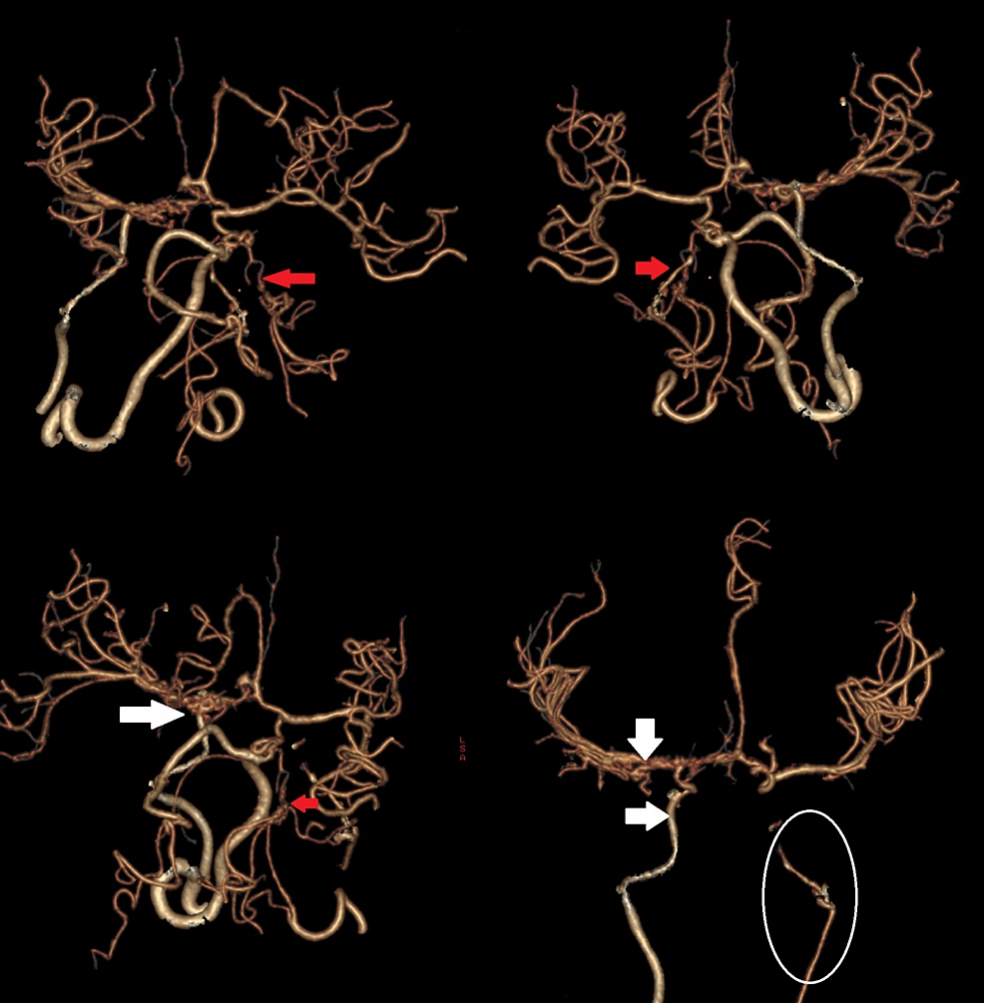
CT angiography (CTA) of the brain reveals stenosis in the cervical, carotid canal, and cavernous part of the right internal carotid artery (white circle), a narrowed left internal carotid artery with collateral vessels in the regions of left M1 and M2 (white arrows), and the right posterior cerebral artery (red arrows)
